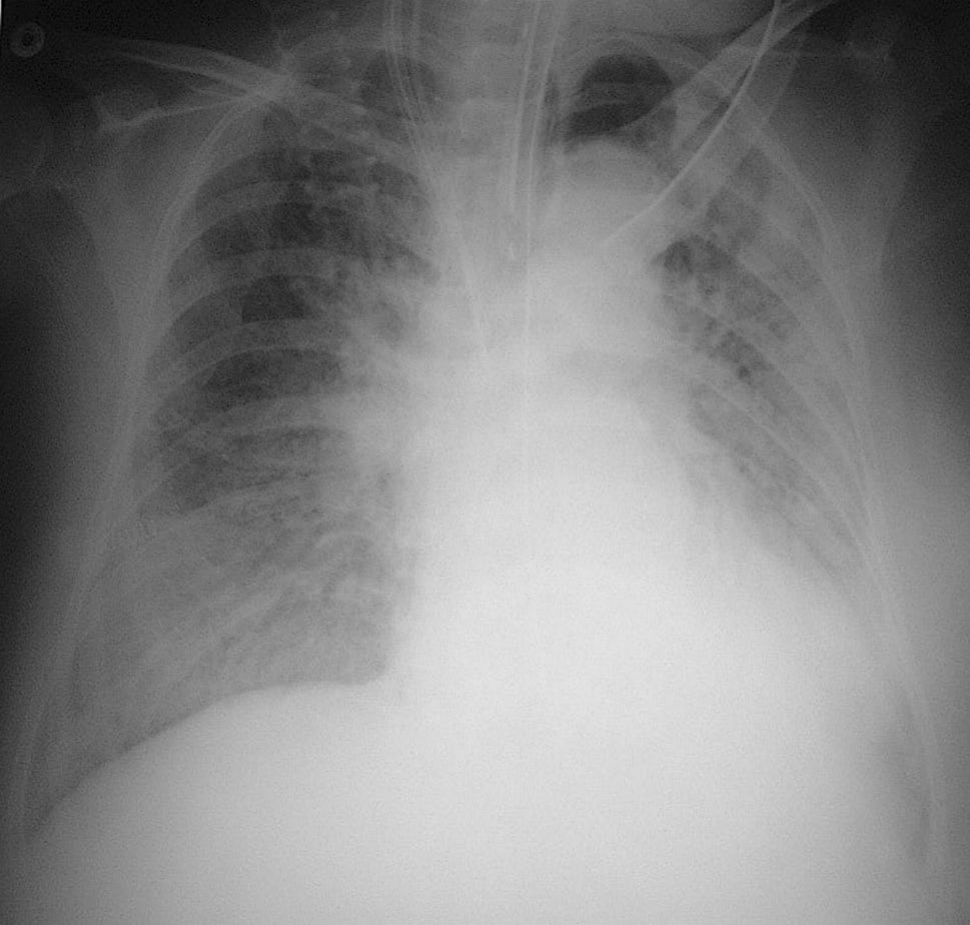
Chest radiograph on day 4, taken immediately after tracheal intubation
